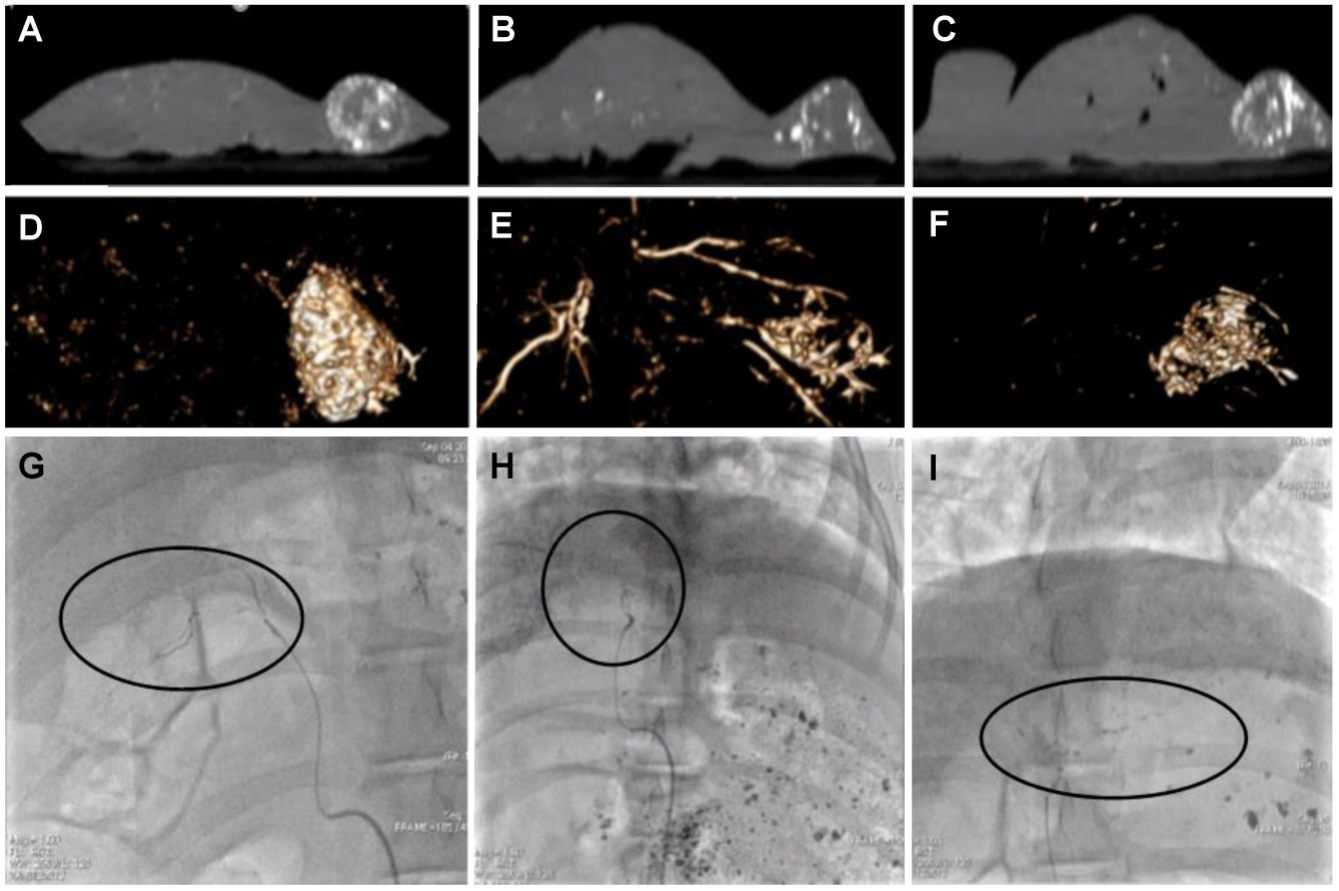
Single plane CT (Discovery IGS 730, GE Healthcare) slices showing emulsion residence after 7 days in VX2; cTACE (A), Non-iodinated (B) and Iodinated (C), with 2D volume reconstruction (D-F). Single representative angiographic images of flow characteristics for cTACE (H), Non-iodinated (G) and Iodinated (I). Black circles highlight flowing emulsions with clear droplet/ “packet-like” emulsions in cTACE (G) and Iodinated (I) but with loose more disperse flow in Non-iodinated (H) echoing flow characteristics observed *in vitro.* Still images extracted from full videos available in S1.
